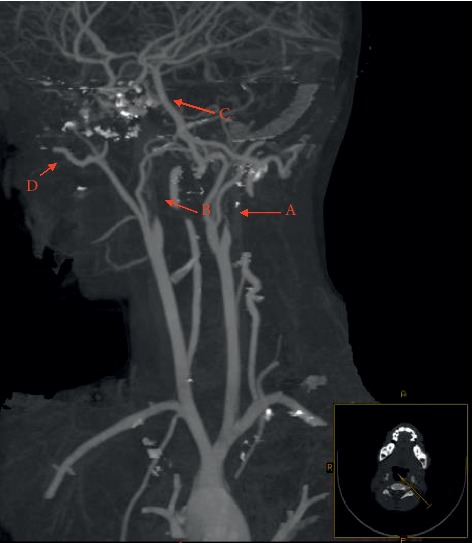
Sagittal view for CT head and neck with arterial phase contrast showed bilateral internal carotid artery (ICA) agenesis as shown by arrows A and B. Arrow C shows collaterals communicating with cerebral circulation that was supplied by an enlarged posterior communicating artery (POCMA) from a basilar artery (BA) and arrow D shows carotid rete mirabile from the external carotid artery.
